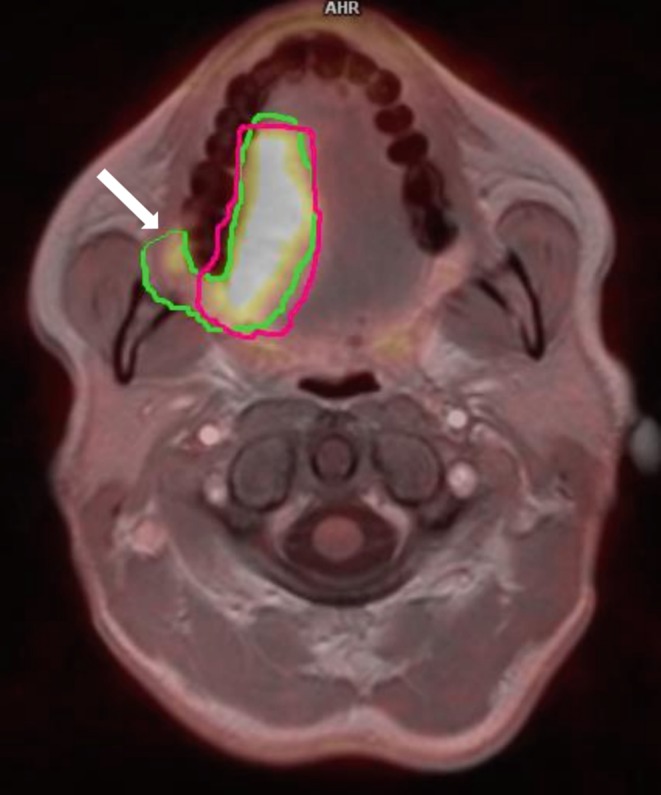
Primary tumor volume (GTV, gross tumor volume) delineated with manual method and presented on fusion of 18-fluorine-labeled fluorodeoxyglucose positron emission tomography (PET) and magnetic resonance (MRI) images. PET-based GTV (*green line*) are larger than MRI-based GTV (*pink line*) and include tumor’s infiltration on the retromandibular triangle (*arrow*)
